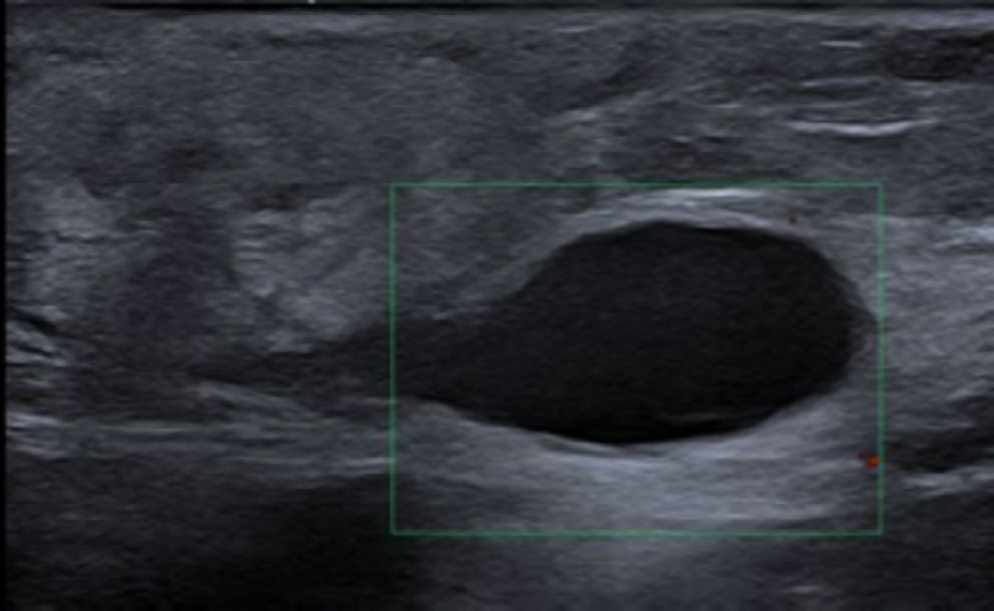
Postoperative seroma detected on HFUS. The image shows a well‐circumscribed, anechoic collection with thin, smooth walls and absent Doppler flow, consistent with sterile serous fluid in the surgical bed. Typical resolution occurs spontaneously or post US‐guided aspiration; the absence of internal echoes/flow helps differentiate it from hematoma or abscess.
